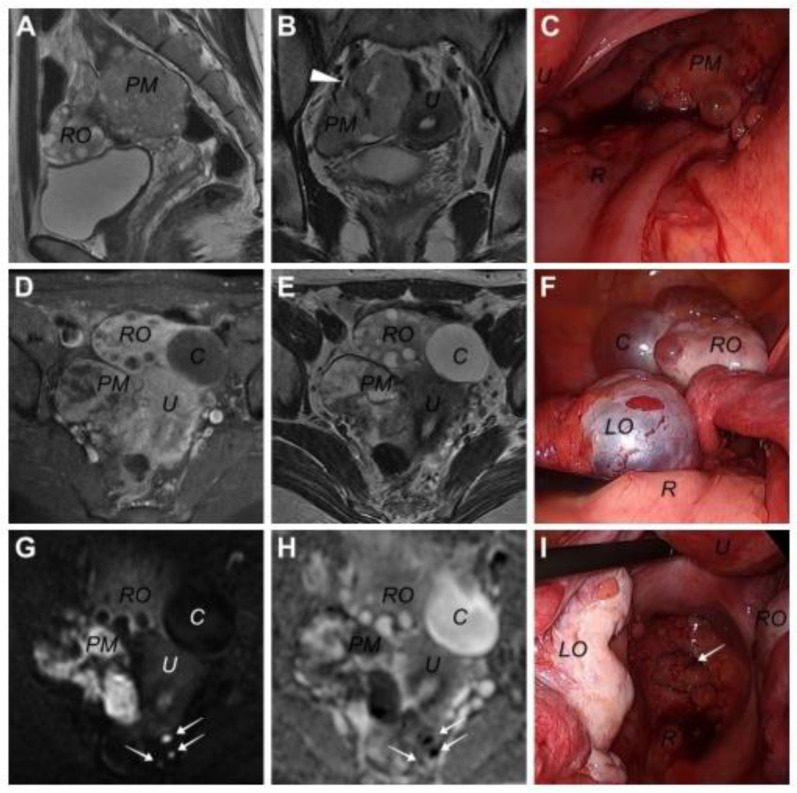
Images of pelvic magnetic resonance imaging and laparoscopic findings in a 31-year-old woman diagnosed with primary solid pseudopapillary neoplasm (SPN) in this case. (A) Sagittal T2-weight image, (B) coronary T2-weighted image, (C) pelvic mass in laparoscopy, (D) contrast-enhanced T1-weighted image, (E) transverse T2-weighted image, (F) both ovaries in laparoscopy, (G) diffusion-weighted image, (H) the ADC map, and (I) metastatic lesions of the peritoneum in laparoscopy. (*PM*: pelvic mass, *RO*: right ovary, *U*: uterus, *R*: rectum, *C*: right ovarian cystic lesion, *LO*: left ovary, arrowhead: right internal iliac artery supplying the pelvic mass, white arrows: metastatic lesions on rectal serosa or peritoneum).
